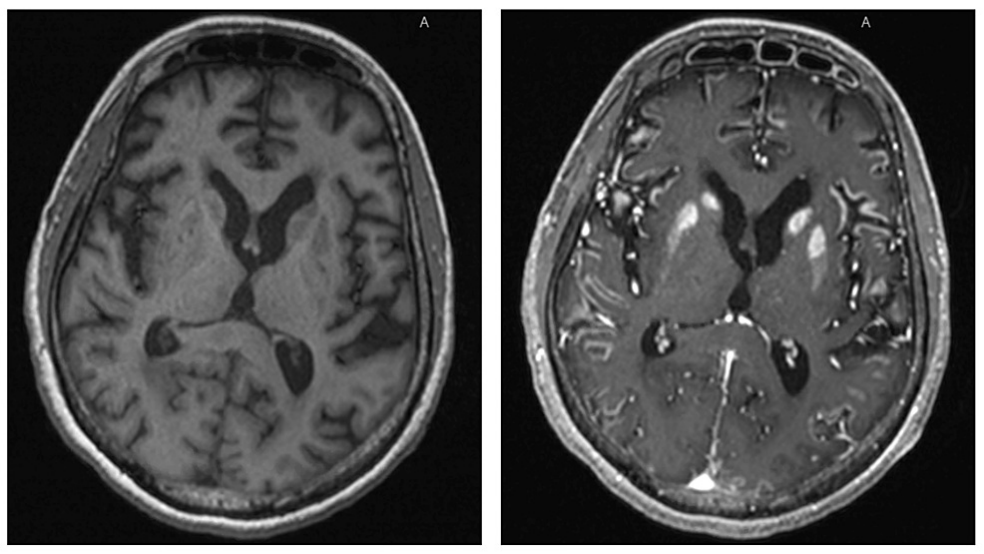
MRI brain scan showing extensive leptomeningeal enhancement and bilateral symmetrical basal ganglia enhancement. T1-weighted image pre-contrast (left) and T1-weighted image post-contrast (right).
